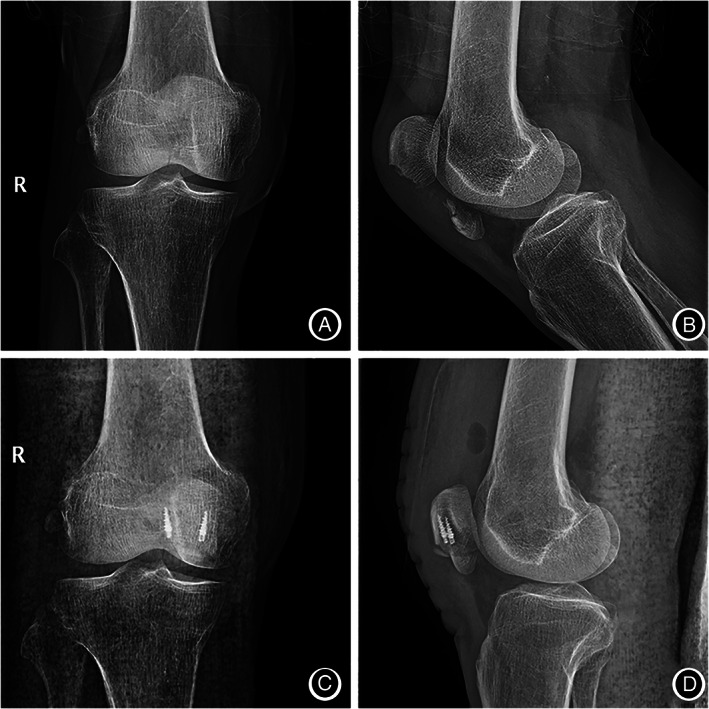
Anterioposterior and lateral knee radiographs of a 55‐year‐old male patient (A,B) Patients with distal pole patellar fractures; (C,D) after treatment with anchor and Krackow‐“8” suture fixation.
